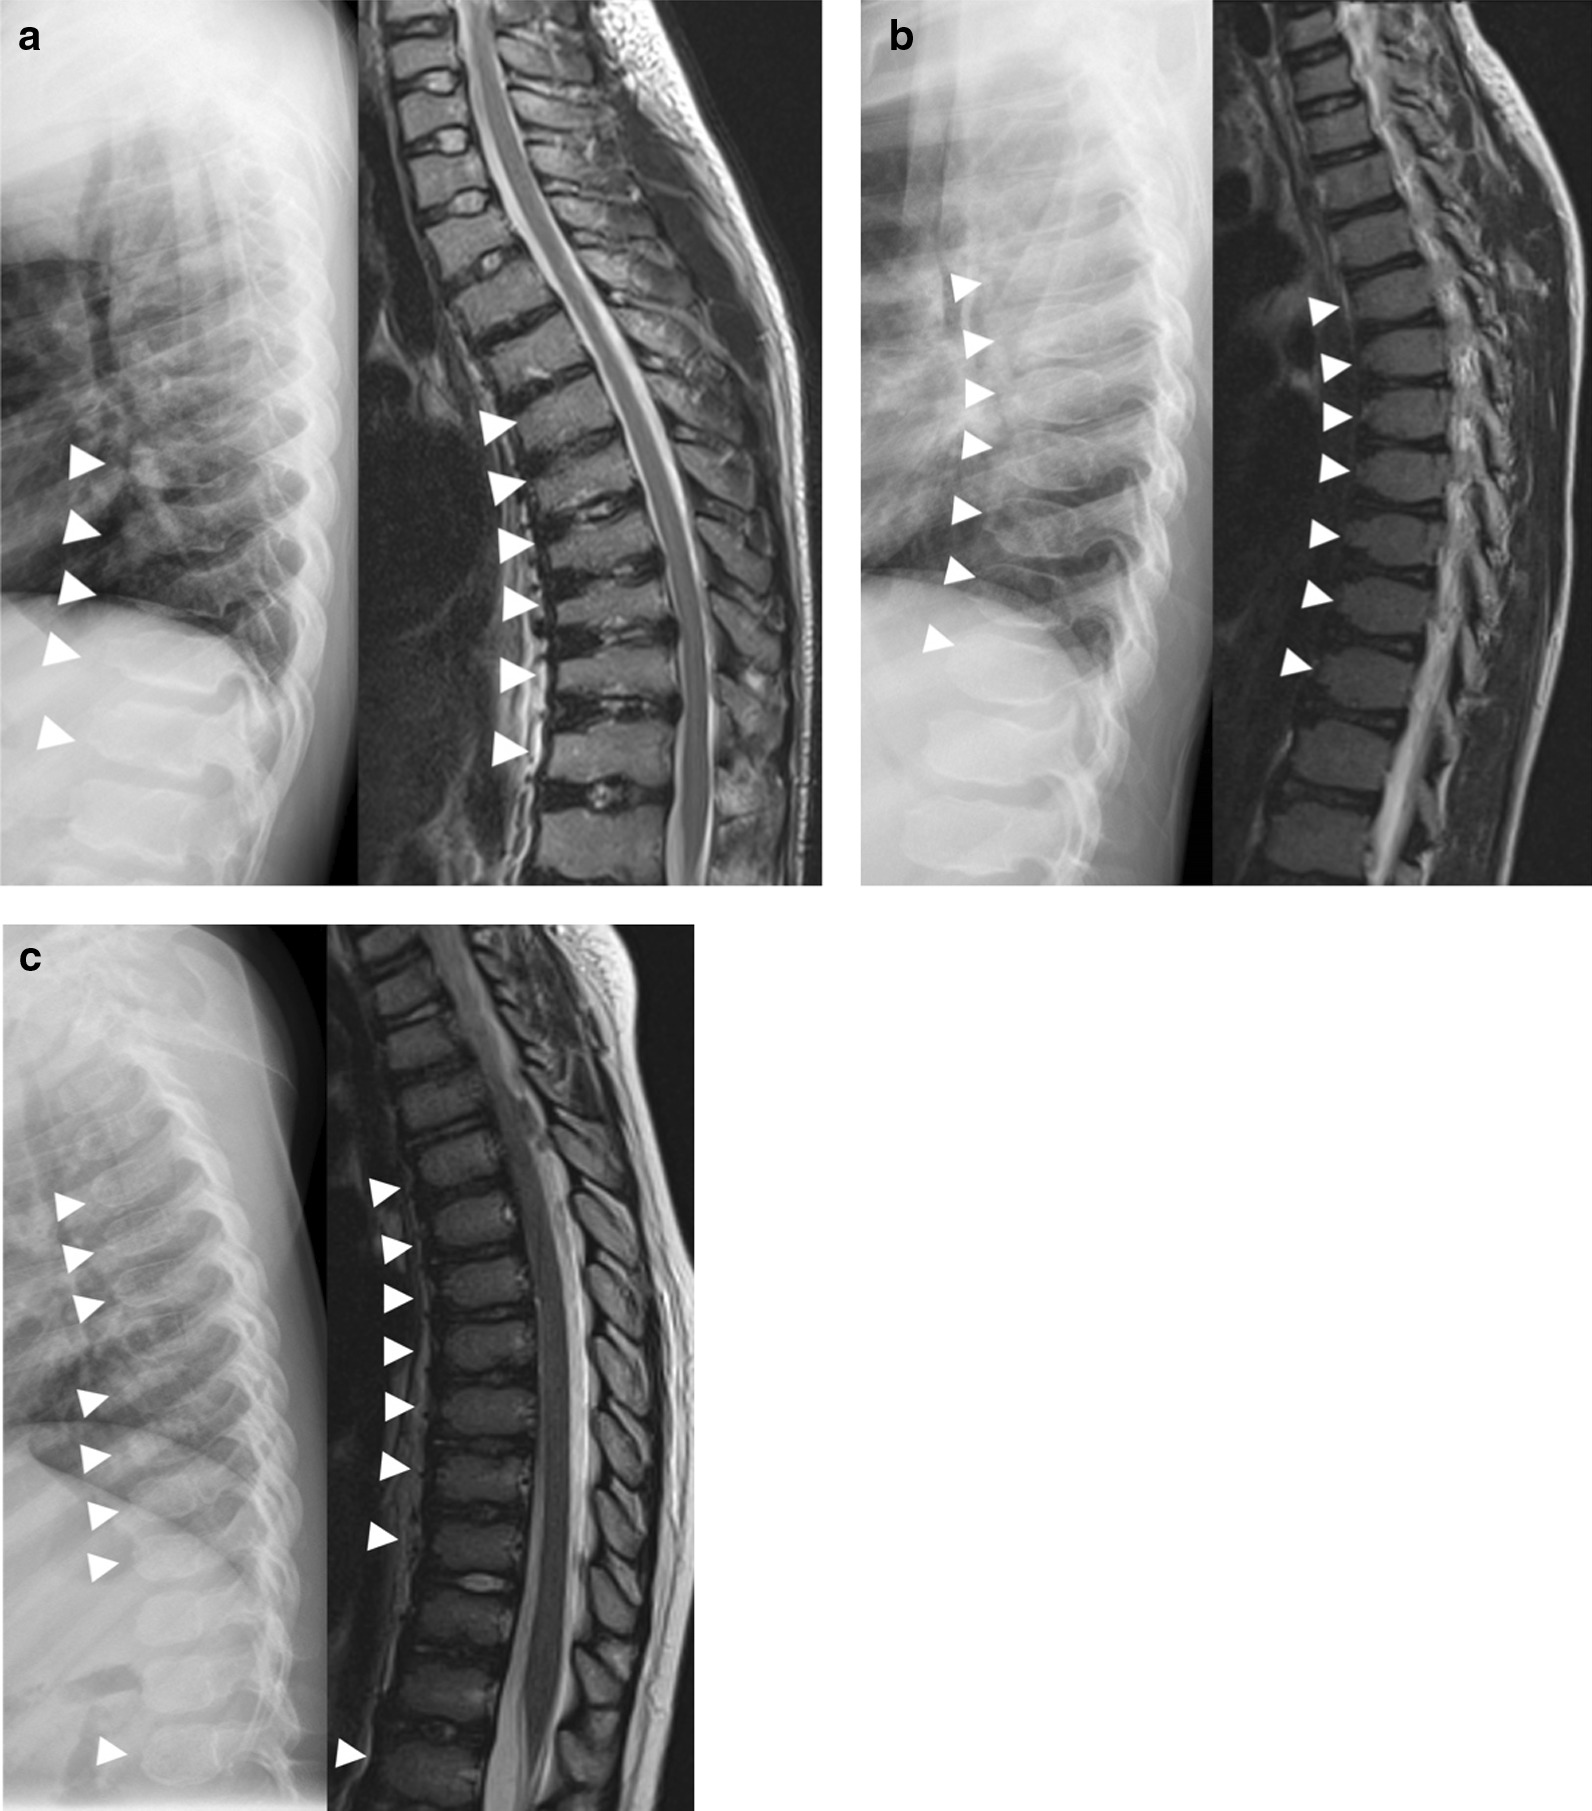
Lateral radiographs and MRIs of the thoracic spine in the three siblings. a In Patient 1, lateral thoracic spine radiograph (left) at 10 years of age shows relatively moderate vertebral body height loss (platyspondyly) and anterior vertebral body beaking, most prominent in the mid-to-lower thoracic spine (arrowheads). Sagittal T2-weighted MRI of the thoracic spine (right) shows moderate platyspondyly with prominent anterior beaking of multiple thoracic and upper lumbar vertebral bodies (arrowheads). The patient was initially diagnosed with spondyloepiphyseal dysplasia on the basis of clinical presentation, platyspondyly, and femoral epiphyseal abnormalities on imaging, although anterior beaking in the middle third of the spine is highly suggestive of MPS IVA, and vertebral flattening is not as prominent as would be expected in spondyloepiphyseal dysplasia. b In Patient 2, lateral thoracic spine radiograph at 6 years of age (left) shows anterior beaking of multiple thoracic vertebral bodies (arrowheads). Sagittal T2-weighted MRI of the thoracic spine at 10 years of age (right) shows anterior vertebral body beaking (arrowheads) with mild vertebral height loss without platyspondyly. Presence of anterior vertebral body beaking in this case is strongly suggestive of MPS IVA. c In Patient 3, lateral thoracic spine radiograph at 3 years of age (left) shows anterior beaking of multiple thoracic vertebral bodies without significant vertebral body height loss. Sagittal T2-weighted MRI of the thoracic spine at 5 years of age (right) highlights anterior vertebral body beaking. As with this patient’s siblings, anterior vertebral body beaking supported the diagnosis of MPS IVA
